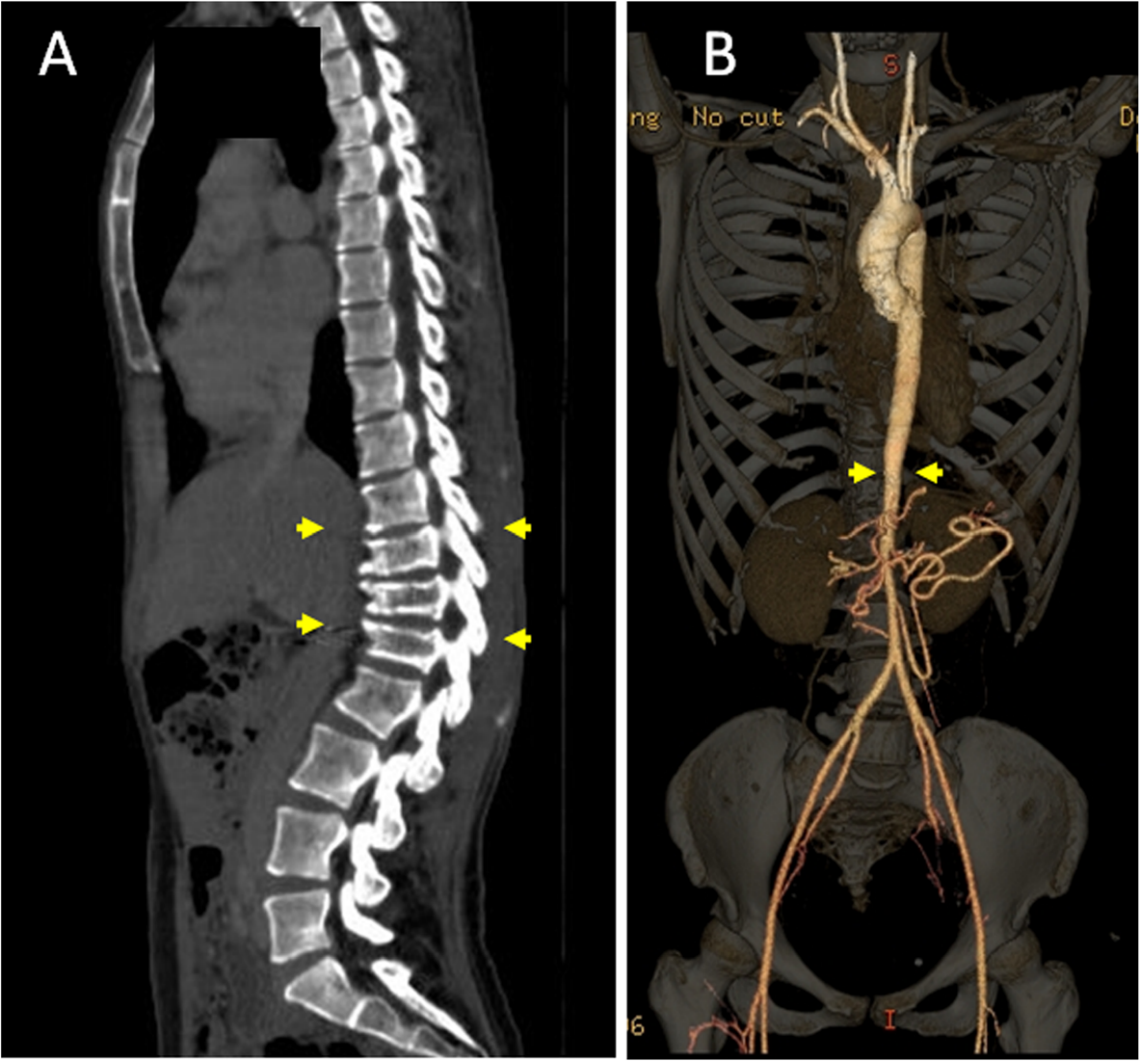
CT scan 28 years after the PBT. (A) Growth retardation in the ventral vertebral body. (B) Narrowing of the aorta below the irradiated field.
